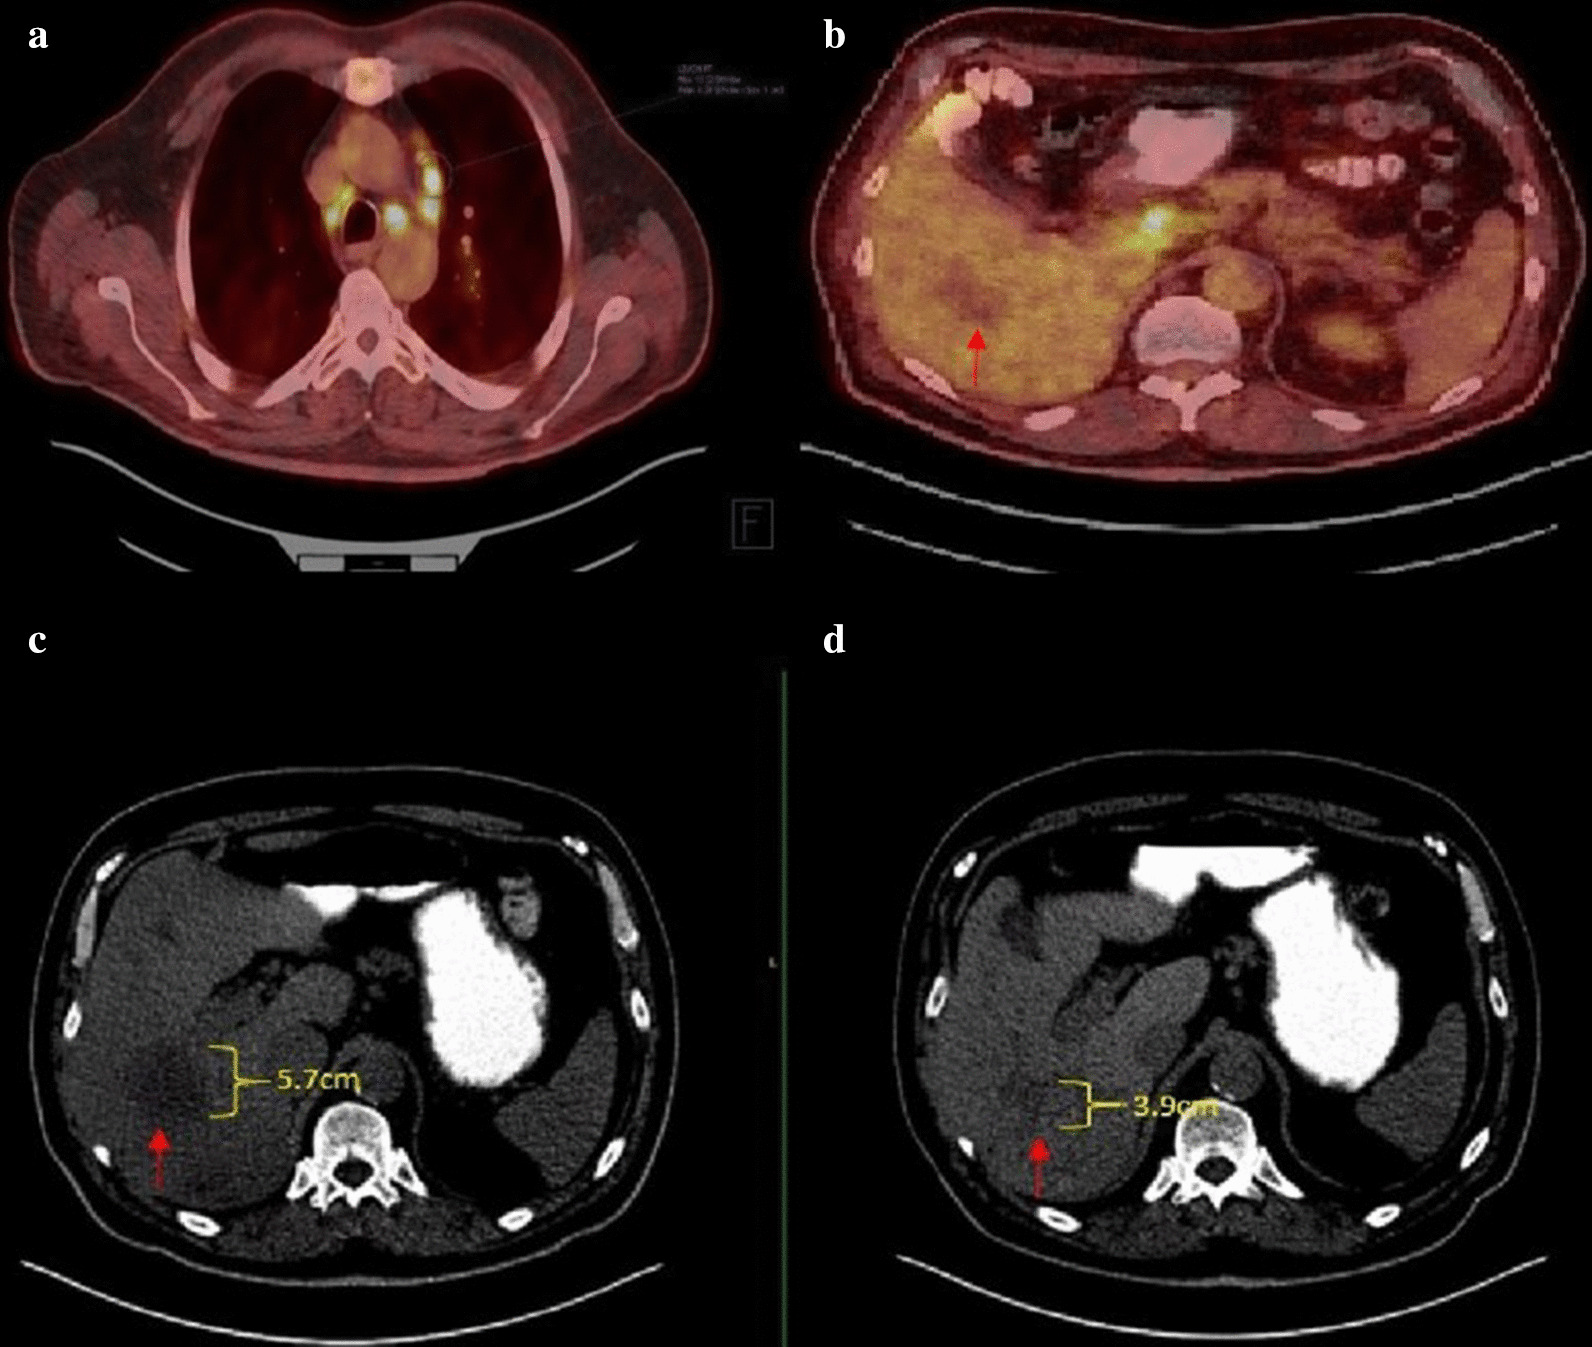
a FDG-PET/CT demonstrating hypermetabolic mediastinal lymph nodes. b FDG-PET/CT showing a hypermetabolic portacaval lymph node, though the known liver metastasis was not FDG-avid (red arrow). Below these PET images, two CT scans of the abdomen & pelvis are shown: one before treatment was initiated (c) and one 6 months after treatment was completed (d). The dominant right liver lesion decreased from 5.7 × 4.4 cm to 3.9 × 3.6 cm in size (red arrow)
